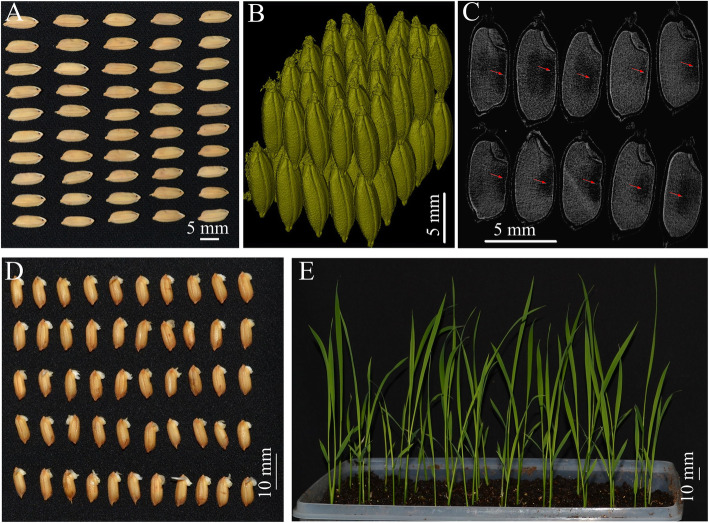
in vivo analysis of rice (Zhenshan 97B) chalkiness. a 50 living mature rice seeds; b Separated and reconstructed 3D image of rice grain; c One of cross-sections of rice grain; d Germination analysis of rice grain after X-ray scanning. e Growth analysis of rice seedlings after X-ray scanning. Dark areas indicated by red arrows represent the location of rice chalkiness. Three independent biological replicates were analyzed
